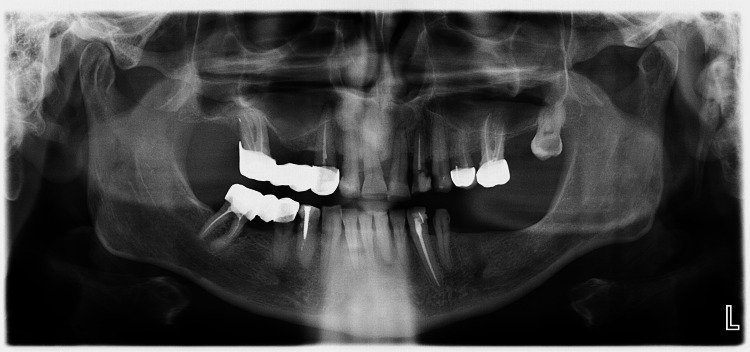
Preoperative panoramic radiograph (Case 1)
